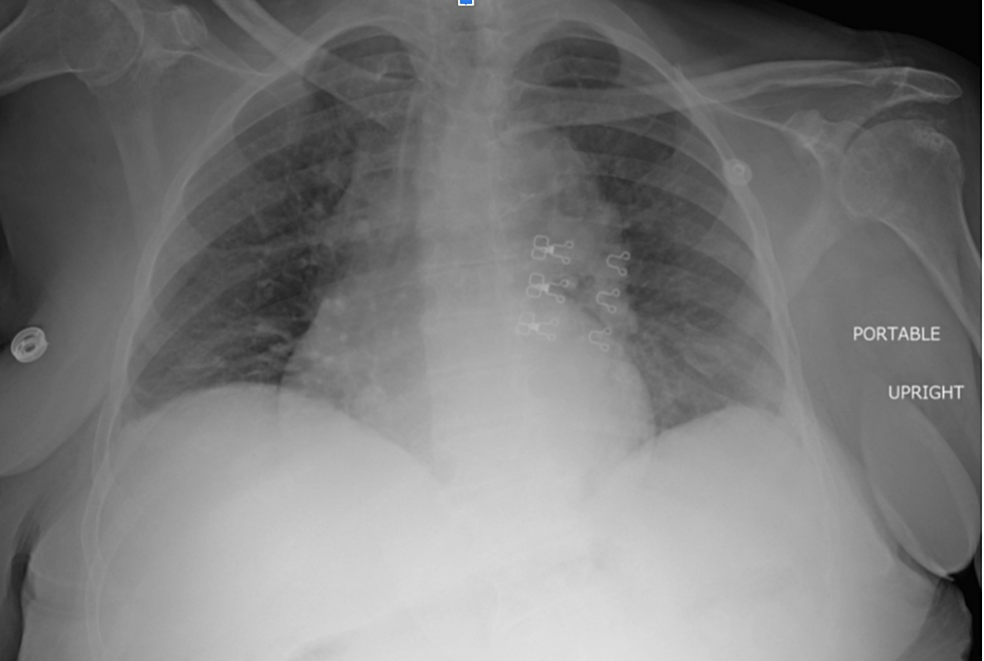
Portable chest X-ray Initial chest X-ray demonstrating diffuse interstitial lung prominence with an elevated hemidiaphragm suggesting low lung inspiration. Moreover, enlarged right atrium, enlarged peripheral hypervascularity, and pruning of the pulmonary vessels can be appreciated
